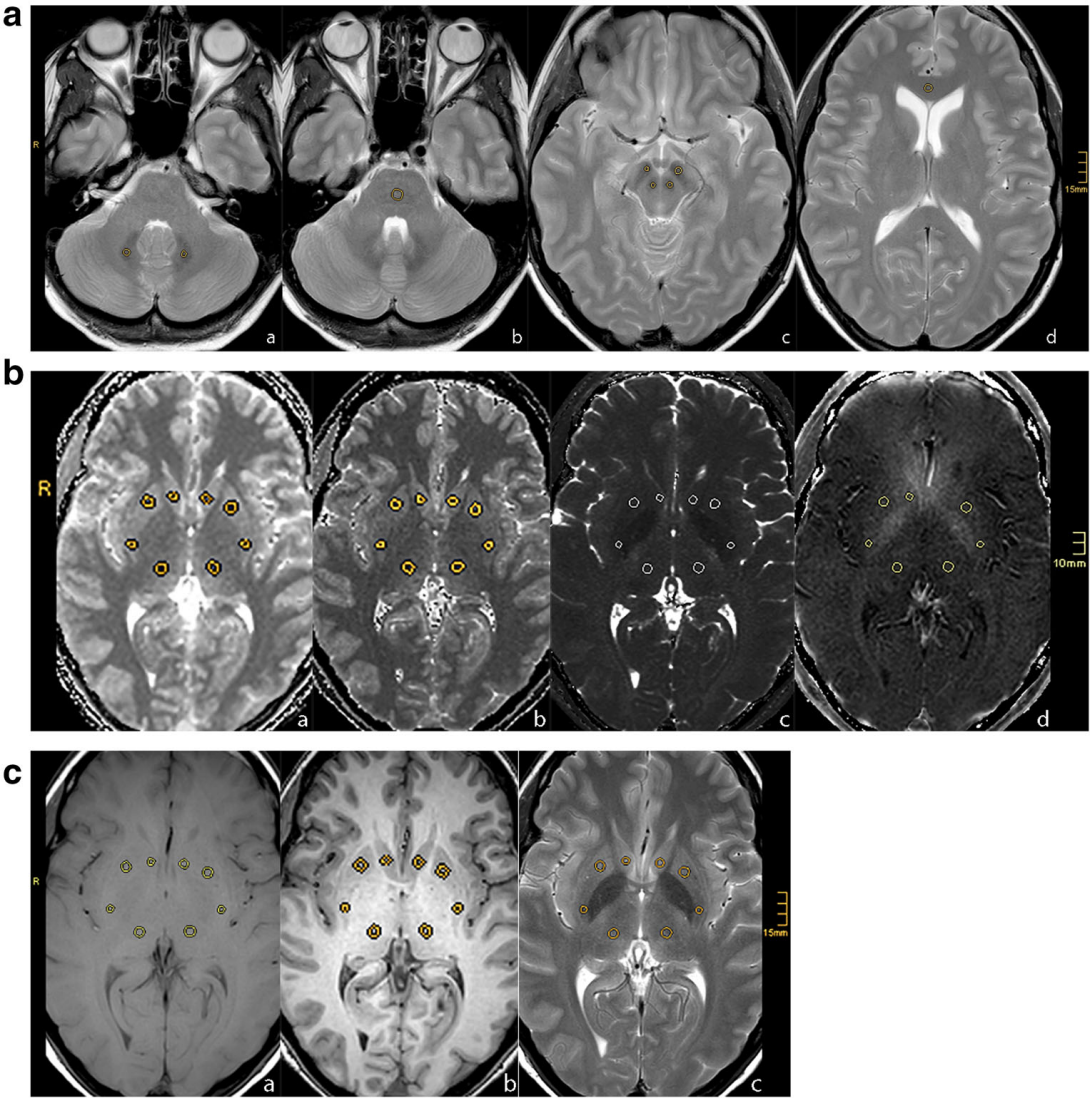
Anatomy-based placement of regions-of-interests (ROI) in a 48-year-old study participant who had received 13 doses of gadolinium-based contrast agents (GBCA) with a cumulative volume of 194 mL in the last 9 years prior to the target brain MRI. a: ROI placements in the dentate nucleus (a), the pons (b), the substantia nigra and the nucleus ruber (c), and in the crus anterior of the internal capsule (d) in the T2wTSE acquisition sequence. b: ROI placements in the globus palidus, the putamen, the caudate nucleus, and the thalamus for the acquisition sequences Look-Locker (LL) T1 mapping (a), variable flip angle (VFA) T1 mapping (b), T2 mapping (c), and R2* mapping (d). c: ROI placements in the globus palidus, the putamen, the caudate nucleus, and the thalamus for the qualitative T1w3D sequence (a), the qualitative T1wSEM (b), and the qualitative T2wTSE sequence
